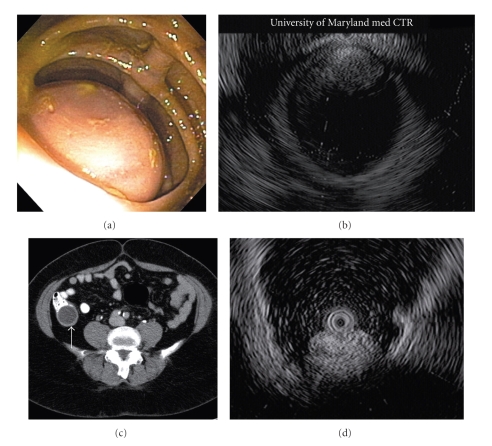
Endoscopic, EUS, and radiographic images of appendiceal findings. (a) Endoscopic image of an appendiceal mucocele; (b) endosonographic image of an appendiceal mucocele; (c) CT scan image of an appendiceal mucocele (arrowhead); (d) endosonographic image of an appendiceal lipoma.
